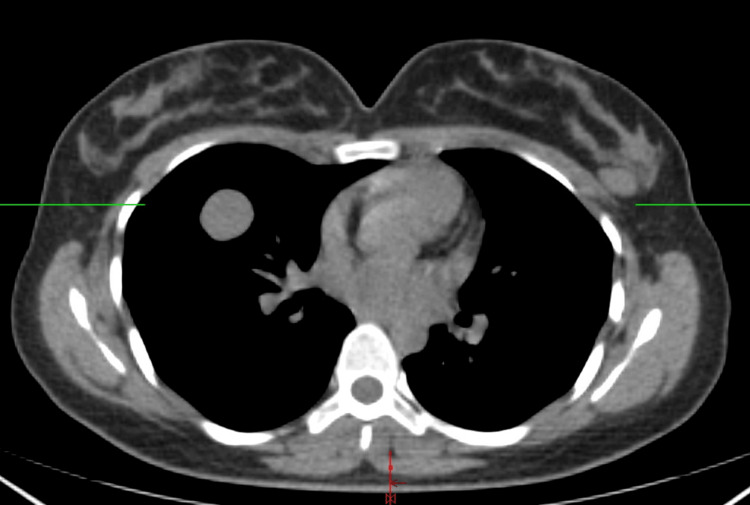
CT scan CT scan of the chest showing a 2.5 cm x 2.6 cm x 2.4 cm round nodule in the right middle lobe with suspected calcification.
